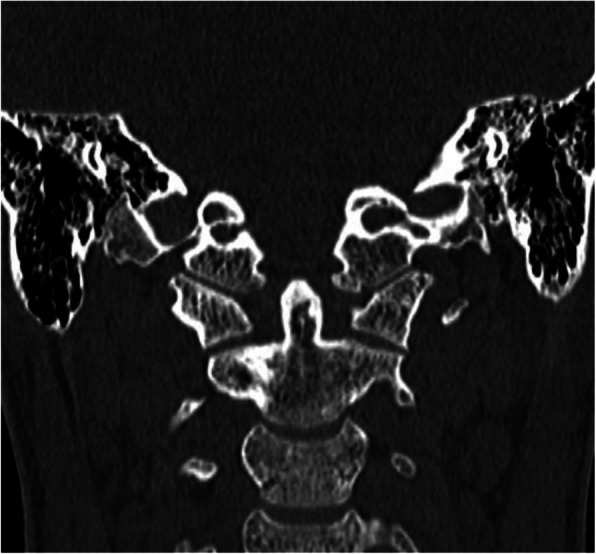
Patient from Figs. 1 and 2. CT performed 5 months after the accident. Treatment—rigid neck collar for 12 weeks
